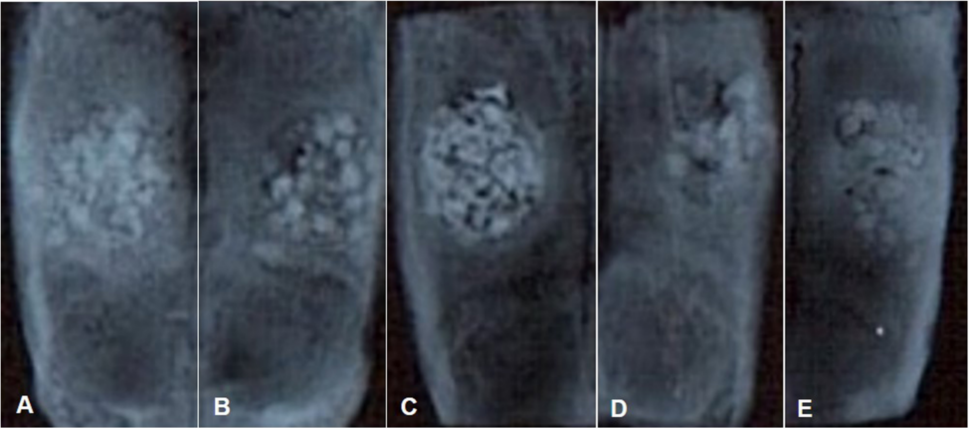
Horizontal radiographic views of the BMP® implanted frontal bone after 8 weeks, no beam treatment (A), 1 MeV-60 kGy (B), 1 MeV-120 kGy (C), 2 MeV-60 kGy (D), and 2 MeV-120 kGy (E).
